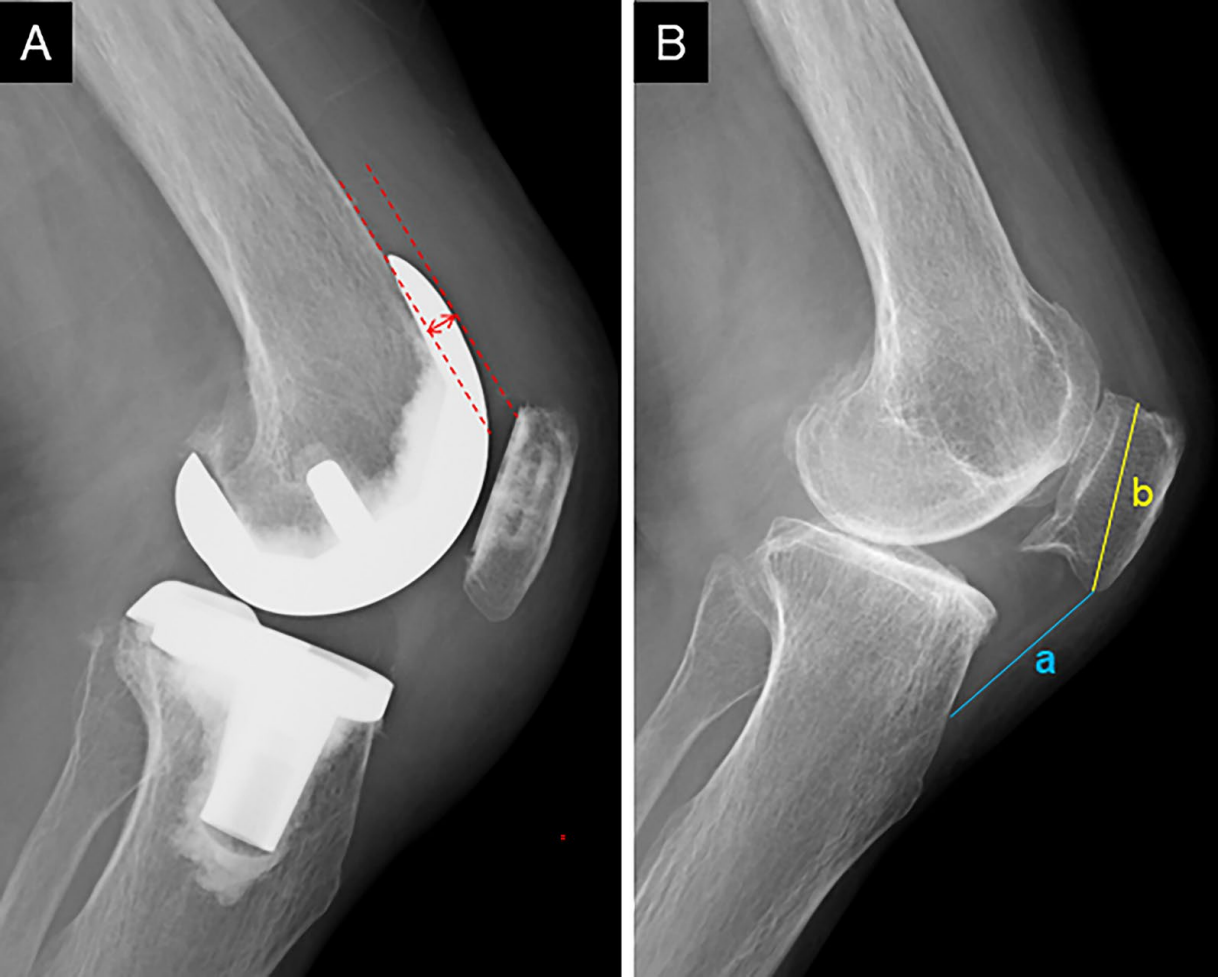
Radiographic measurement using a lateral knee radiograph. A AFO was defined as the distance between the anterior femoral cortex and the anterior aspect of the femoral condyle or femoral component (red double-headed arrow). B ISR was defined as the ratio of the distance between the distal pole of the patella and the tibial tuberosity (a) to the distance between the distal and proximal poles of the patella (b). *AFO* anterior femoral offset, *ISR* Insall–Salvati ratio
